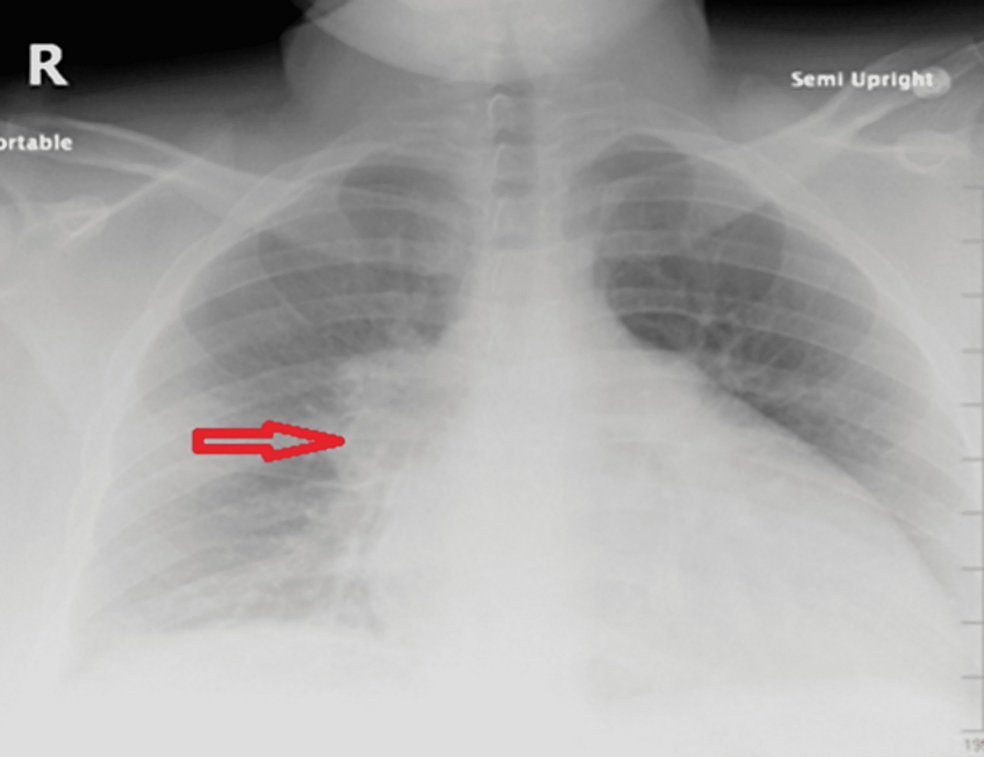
CXR showing a right hilar mass (horizontal red arrow) CXR: chest X-ray
